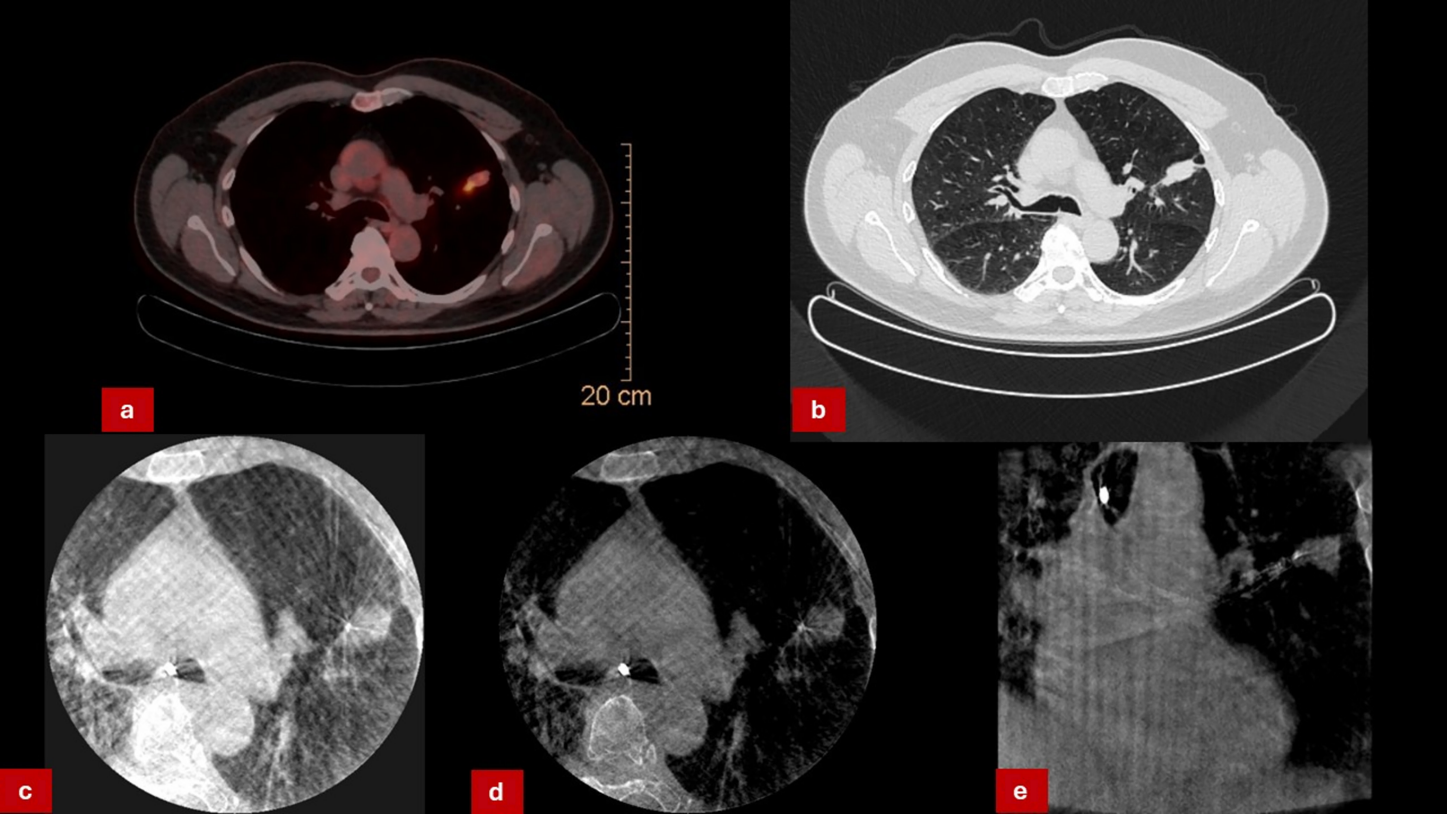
(a) PET‐CT scan of suspicious left upper lobe lesion. (b) CT‐scan showing suspicion of lung cancer in the left lung. (c–e) CBCT‐Imaging showing “Tool‐in‐lesion” (1.1 mm cryoprobe placed within suspicious lesion).
